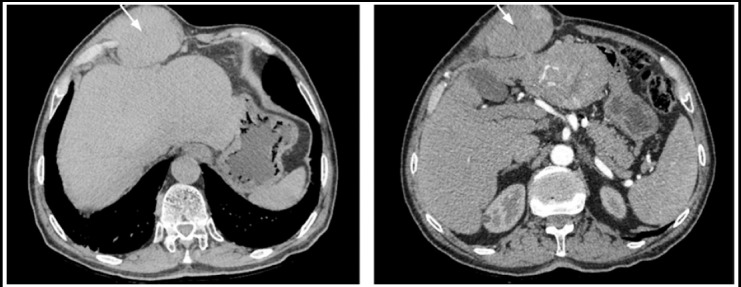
Abdominal computed tomography, hypervascular, solid, non-homogenous mass of 10 X 7 X 12 cm size adjacent to thoracic abdominal wall in hepatic left lobe, segment 4.
